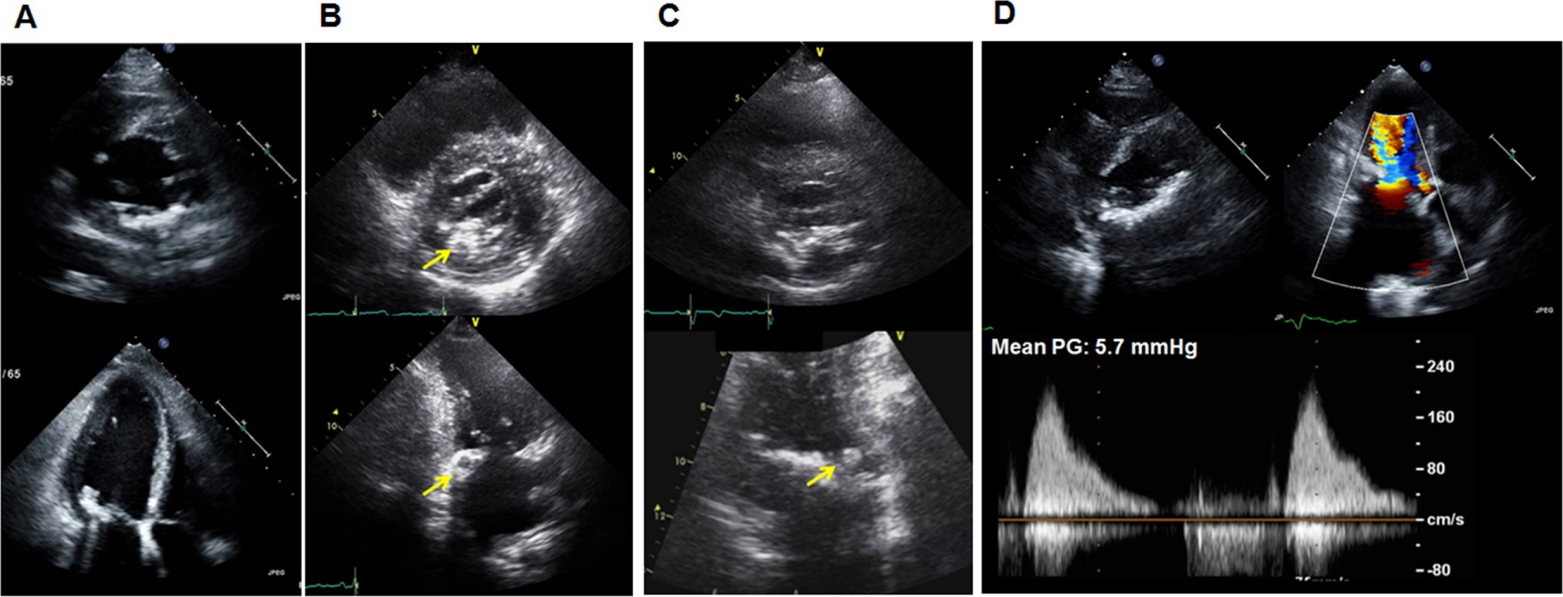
Representative cases. A) MAC in a patient without previous history of stroke: MAC is encircling the posterior mitral annulus without a mobile component, caseous necrosis, or functional stenosis. The MAC score of the patient is 0. B) MAC in a patient with previous history of stroke: Extent of MAC is similar to that in patient A; however, caseous necrosis of MAC (large, round, echodense mass with central areas of echoucencies) is noted at the posterior mitral annulus. The MAC score of the patient is 1. The arrow indicates caseous necrosis of MAC. C) MAC in a patient with previous of history of stroke: MAC is encircling the posterior annulus and has an attached mobile component. The MAC score of the patient is 1. The arrow indicates mobile components of MAC. D) MAC in a patient with previous of history of stroke: Flow acceleration is observed across the MV, and MAC is the encircling posterior annulus. Doppler image shows increased MDPG (5.7 mmHg, HR: 50 bpm). The MAC score of the patient is 1.
